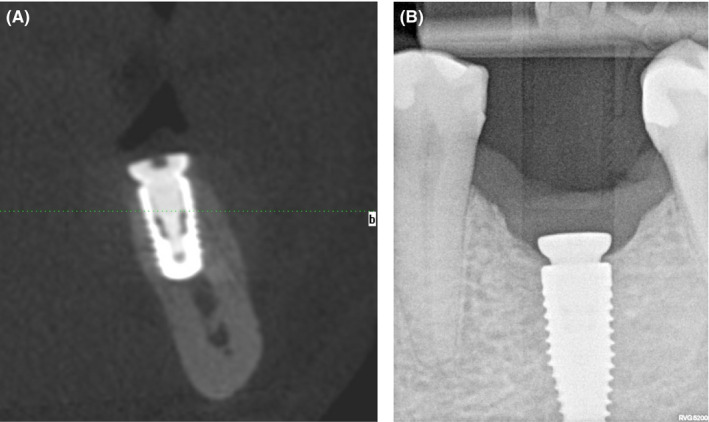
A, Cross‐section CBCT image after 6 mo; (B) Periapical radiograph after 6 mo
